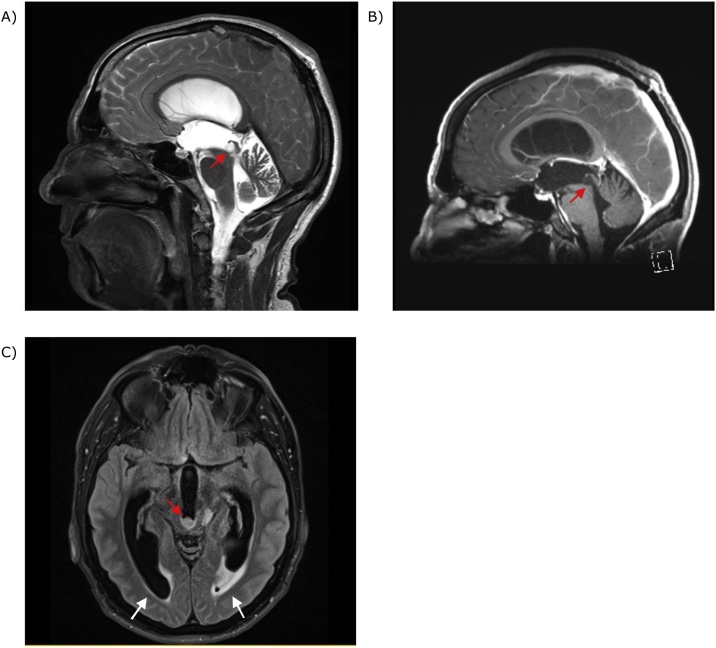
MRI brain showing: A - Sagittal T2 sequence with posterior third ventricular lesion obstructing aqueduct (arrow); B – Same lesion (arrow) appearing hypointense and non-enhancing on sagittal T1 with contrast sequence; C – Axial FLAIR sequence with lesion appearing hyperintense (red arrow). Obstructive hydrocephalus with prominent temporal horns and transependymal flow (white arrows).
